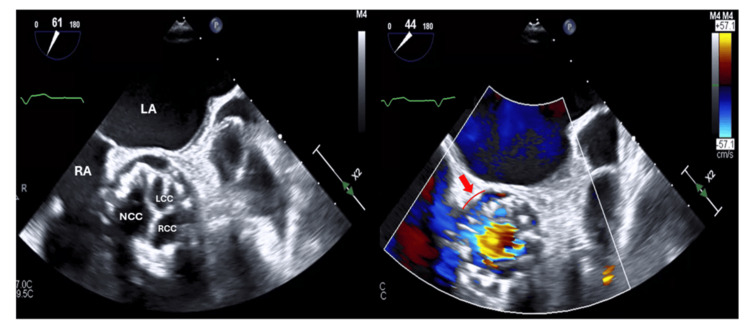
Transesophageal echocardiographic assessment of the bioprosthetic aortic valve with a mild perivalvular leak Transesophageal echocardiogram (ME AV SAX view) in B-mode (left) and with color Doppler (right) of the bioprosthetic valve (BiP-AV) with a demonstration of a mild perivalvular leak (right, red arrow). LA: left atrium, RA: right atrium, NCC: non-coronary cusp BiP-AV, LCC: left coronary cusp BiP-AV, RCC: right coronary cusp BiP-AV
